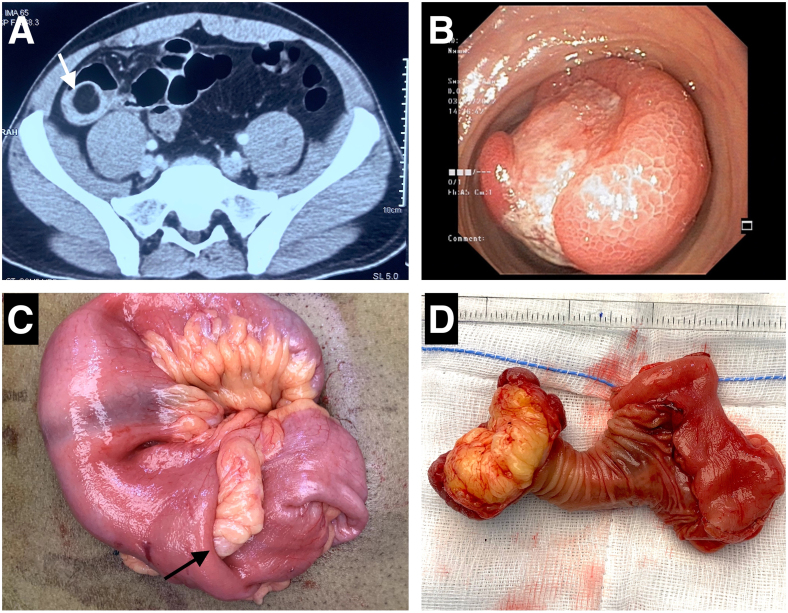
(a) CT scan of abdomen showing an ileal lipoma with ileo-ileal intussusception (arrow). (b) Enteroscopy showing a large subserosal lesion with ulcerated mucosa. (c) Intraoperative finding suggested an ileo-ileal intussusception (arrow) with lipoma as the lead point. (d) Gross examination revealed lipomatous lesion with elongated stalk arising from ileum.
